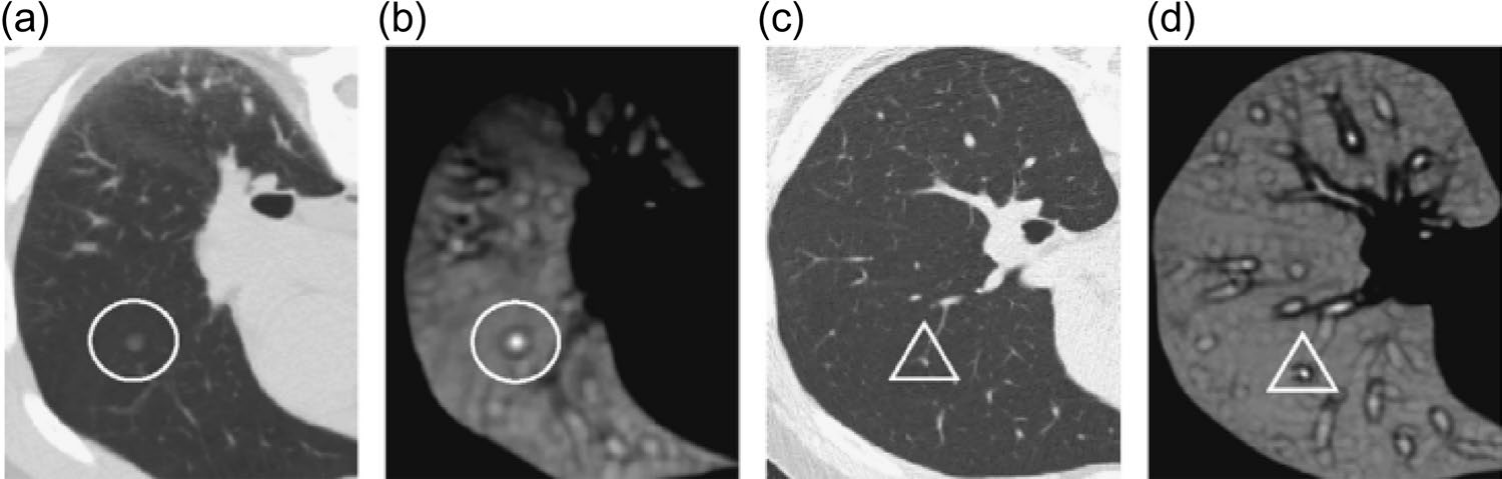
CT scan images show lung nodules with different locations and shapes in CT.
